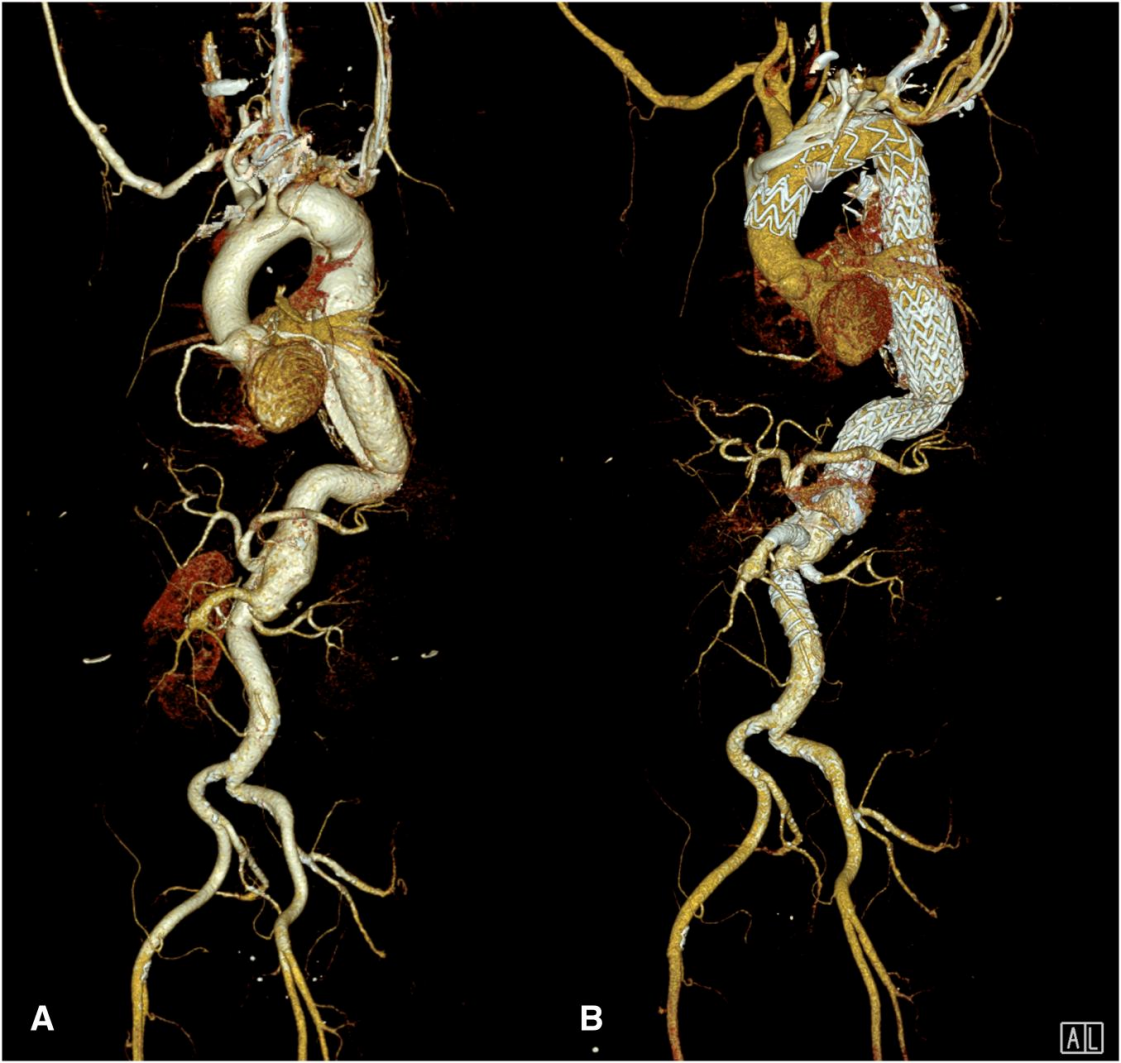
CT 3 D Volume Rendering Before Treatment (A) and After Treatment (B).
